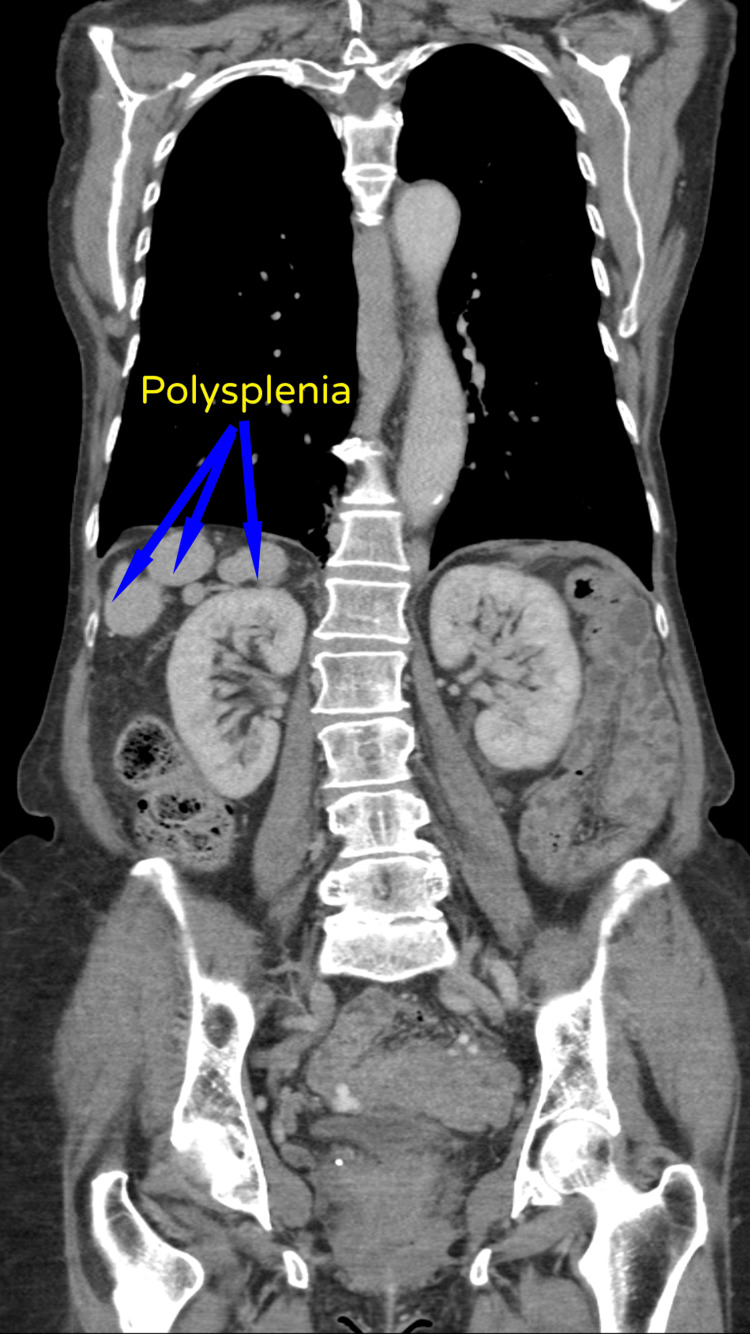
CT-TAP showing polysplenia on the right-hand side CT-TAP: computerized tomography scan of the thorax, abdomen, and pelvis
